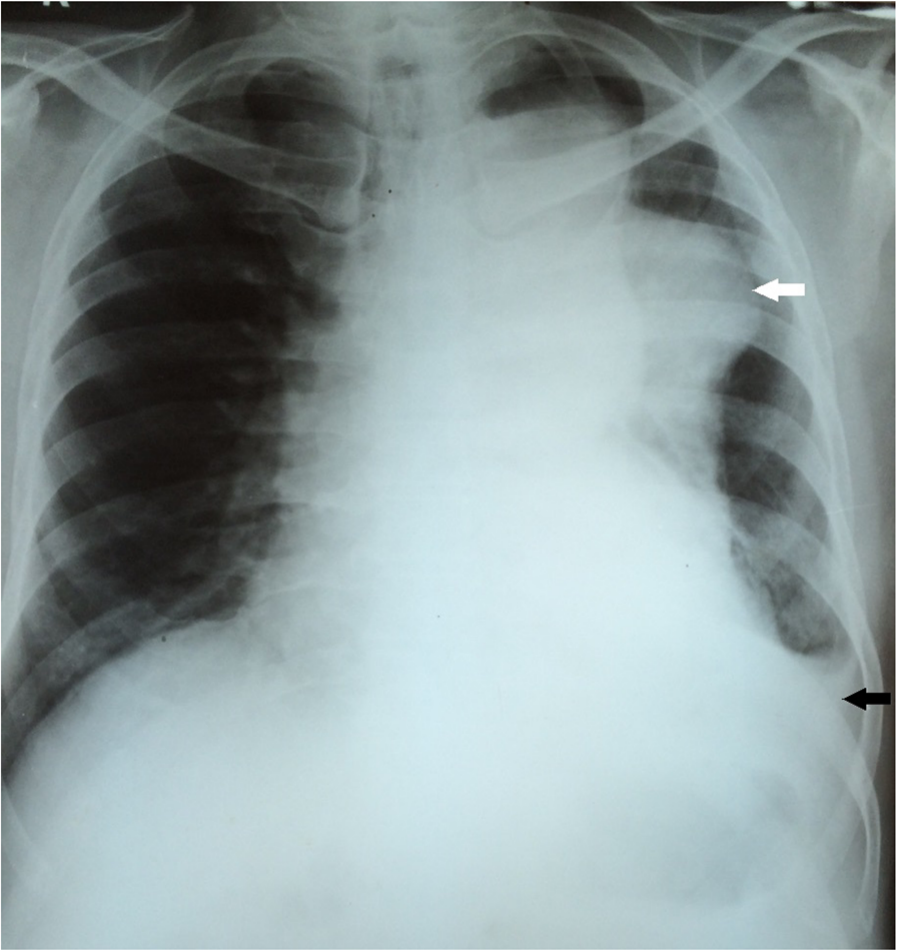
Chest X-ray showing a left side mediastinal mass. A homogeneous, soft tissue, dense, well-defined lesion in the left lung (white arrow) extending from the aortic knuckle to the left hemidiaphragm. Its medial margin merges with the mediastinum and there is a left-sided mild to moderate pleural effusion (black arrow)
